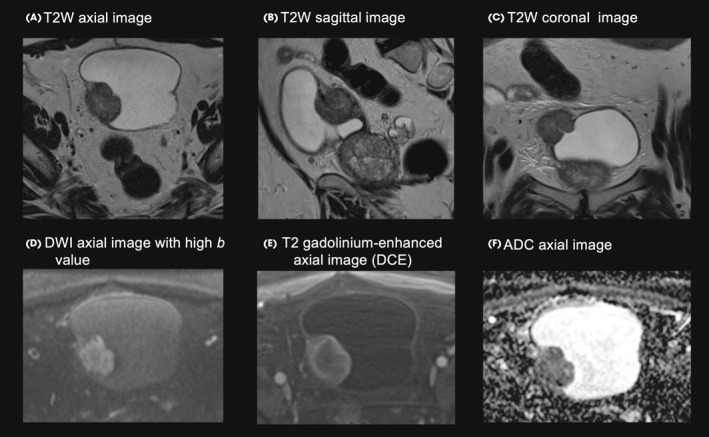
T2W, DWI, DCE and ADC mpMRI image sequences of a large VI‐RADS 4 bladder tumour. ADC, apparent diffusion coefficient; DCE, dynamic contrast‐enhanced imaging; DWI, diffusion‐weighted imaging; T2W, T2‐weighted imaging.
